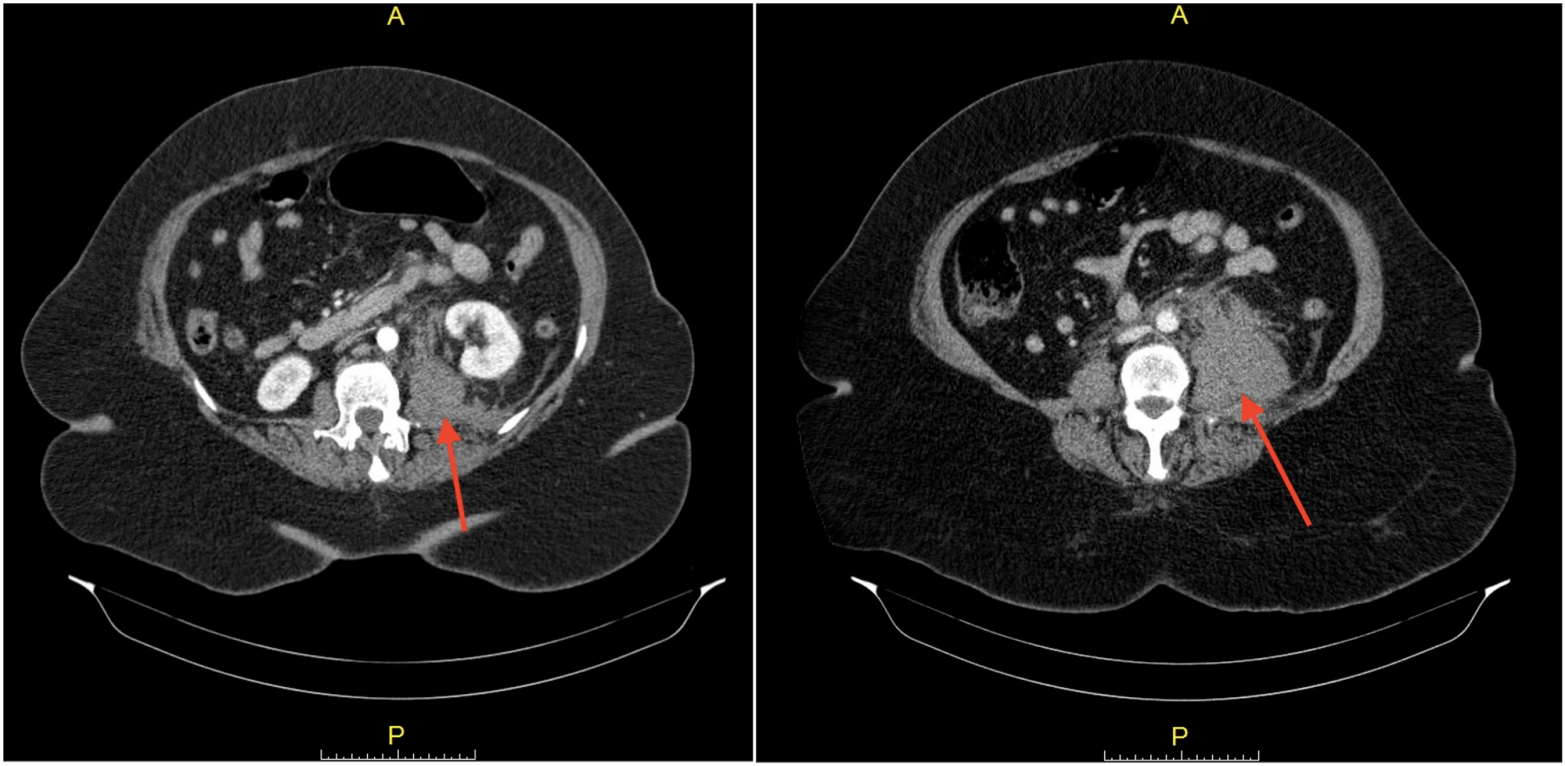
CT angiogram showing a large retroperitoneal hematoma (red arrows) in the left hemipelvis near the origin of the left internal iliac artery and extending along the left iliopsoas muscle and displacing the left kidney anteriorly.
